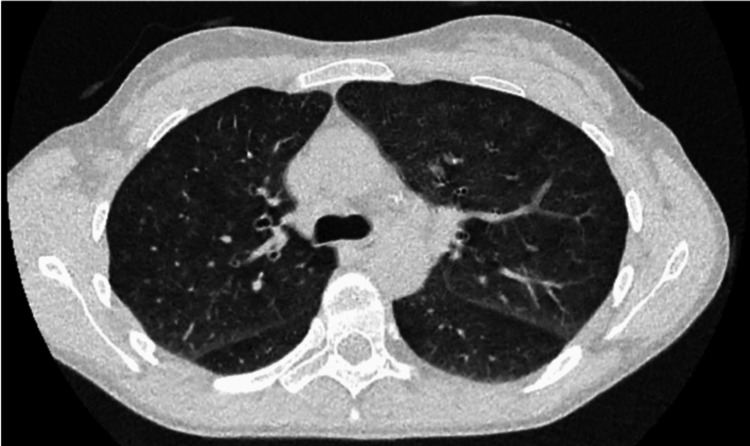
Chest CT scan showing diffuse ground glass opacities.
